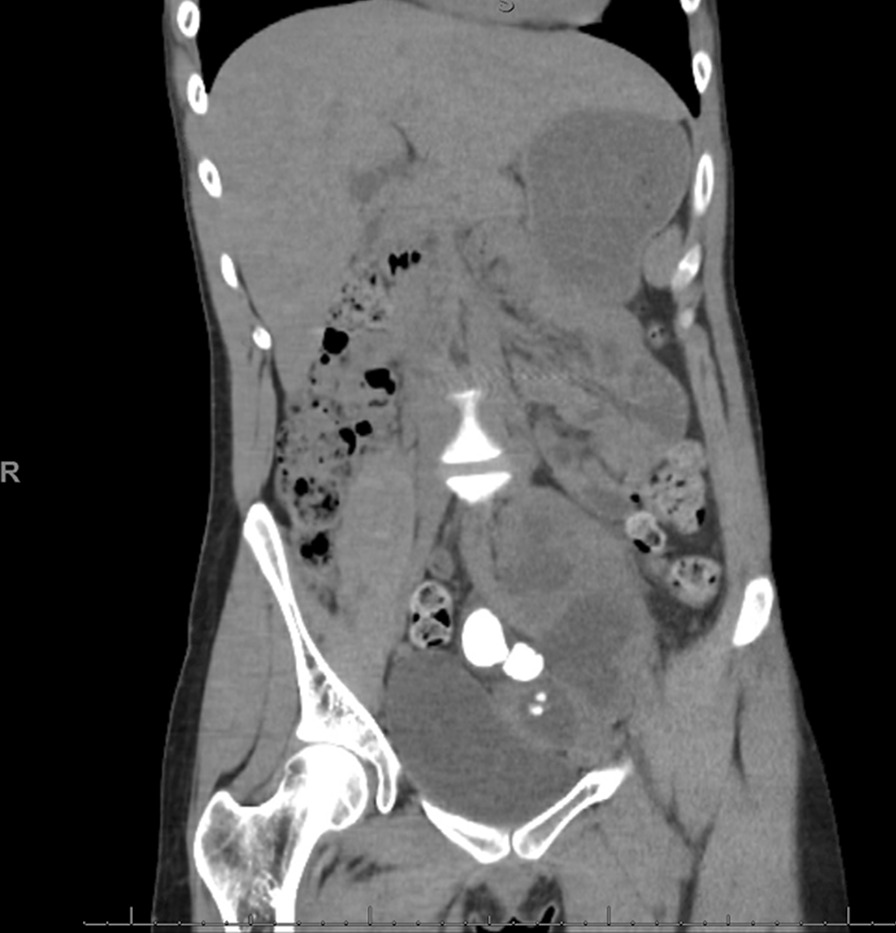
Coronal slice of a computed tomography abdomen/pelvis showing a large stone burden in a solitary pelvic kidney
